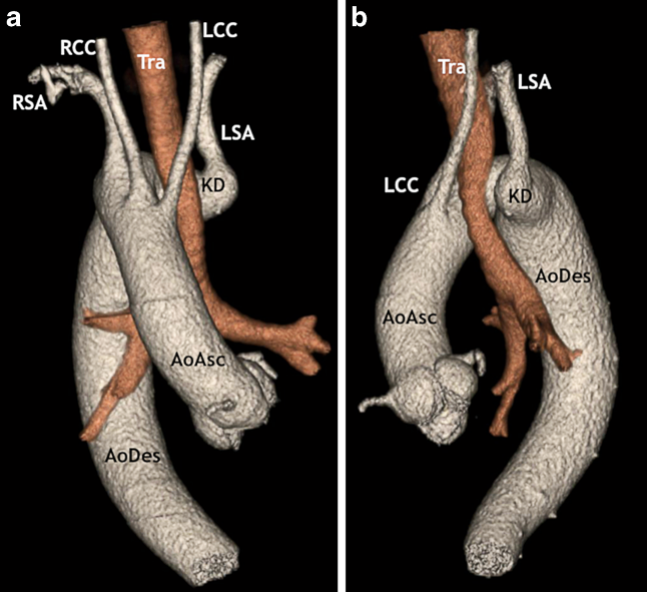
A three-dimensional reconstruction of the CT angiography showing the frontal view (a) and the left lateral view (b) of the right-sided aortic arch giving rise to the aberrant left subclavian artery from the Kommerell diverticulum. (*AoAsc* ascending aorta, *AoDes* descending aorta, *Tra* trachea, *LCC* left common carotid artery, *RCC* right common carotid artery, *RSA* right subclavian artery, *LSA* aberrant left subclavian artery, *KD* Kommerell diverticulum)
